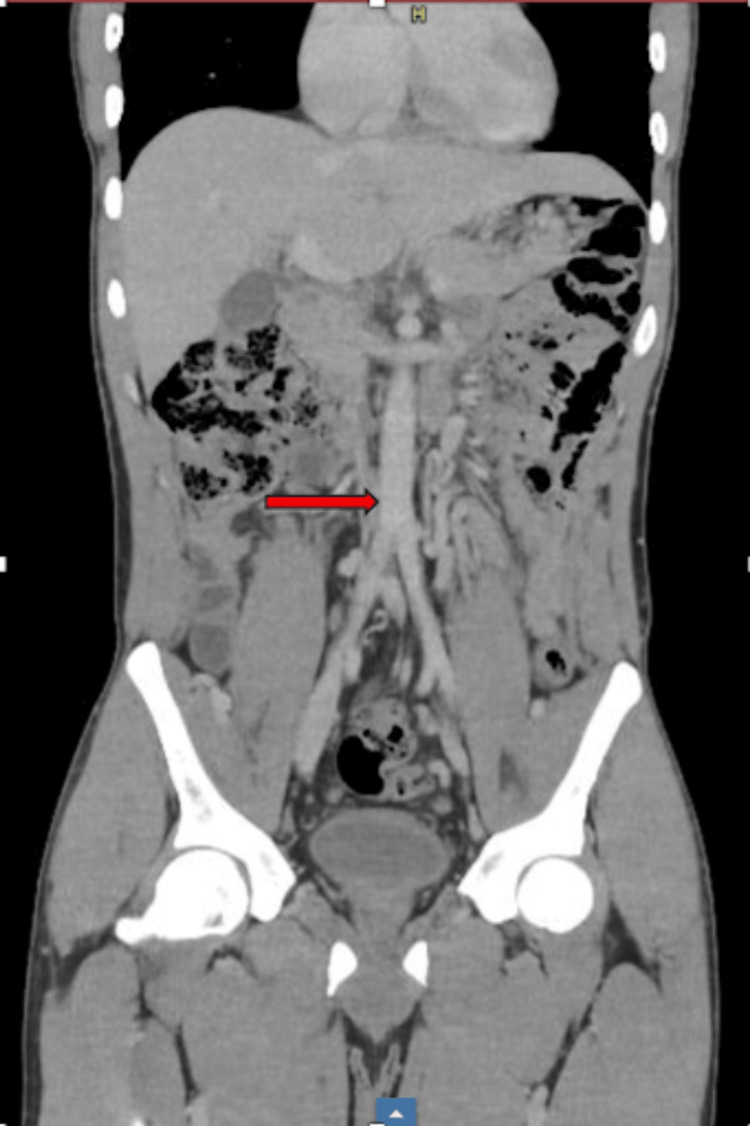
Coronal CT image of the abdomen and pelvis with contrast. The inferior vena cava (IVC) is not visualized along its expected anatomical course to the right of the aorta (red arrow).
